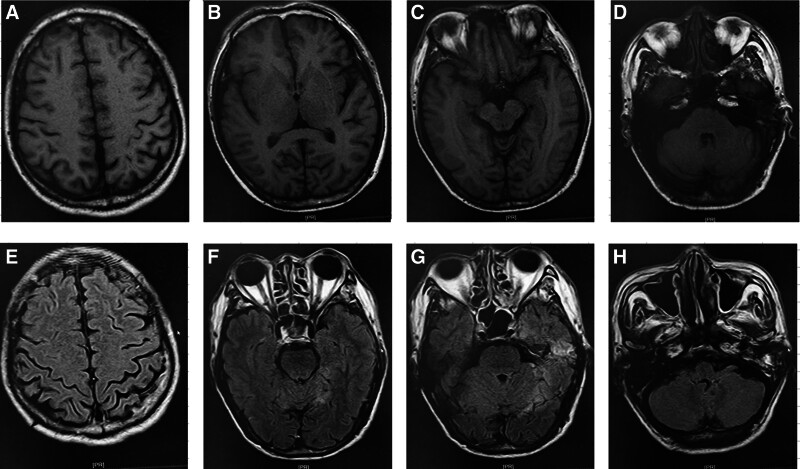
The alterations in the patient head MRI. (A–E) the alternations of the patient nuclear magnetic resonance (MRI) upon admission; (H–J) the alternations of the patient head Computer tomography (CT) 6 months after discharge.
